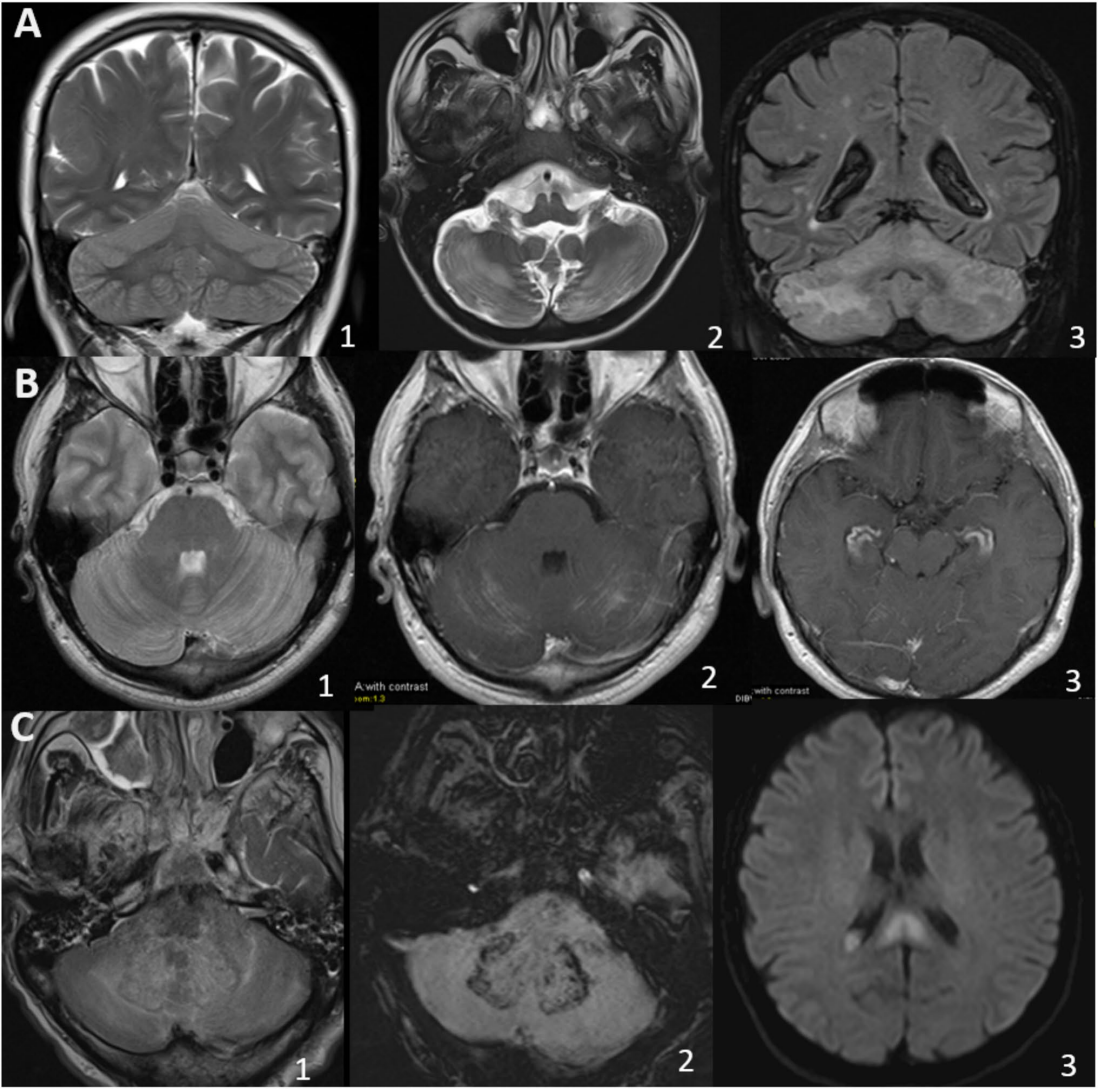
A-C Magnetic Resonance Imaging patterns of cerebellitis. Coronal and axial T2W images demonstrate involvement of the cortex only in Patient 1 with postinfectious cerebellitis (A1), predominantly cortex involvement in Patient 5 with postinfectious SARS-CoV2 cerebellitis (A2) and coronal FLAIR shows white matter predominantly involvement in Patient 15 with familial hemophagocytic lymphohistiocytosis (A3). Axial T2W image of Patient 3 reveals symmetrical cortical involvement (B1) and cortical contrast enhancement oriented parallel to the folia (B2). Notably, the same patient also exhibits bilateral hippocampal contrast enhancement (B3). Axial FLAIR (C1) and susceptibility-weighted image (C2) image of a patient with hemophagocytic lymphohistiocytosis demonstrate bilateral involvement of the dentate nuclei, and susceptibility-weighted image reveals hemorrhage within the dentate nuclei. Diffusion-weighted imaging of Patient 1 reveals a focal restricted diffusion in the splenium of the corpus callosum (C3). This finding is consistent with mild encephalopathy with reversible splenial lesion
